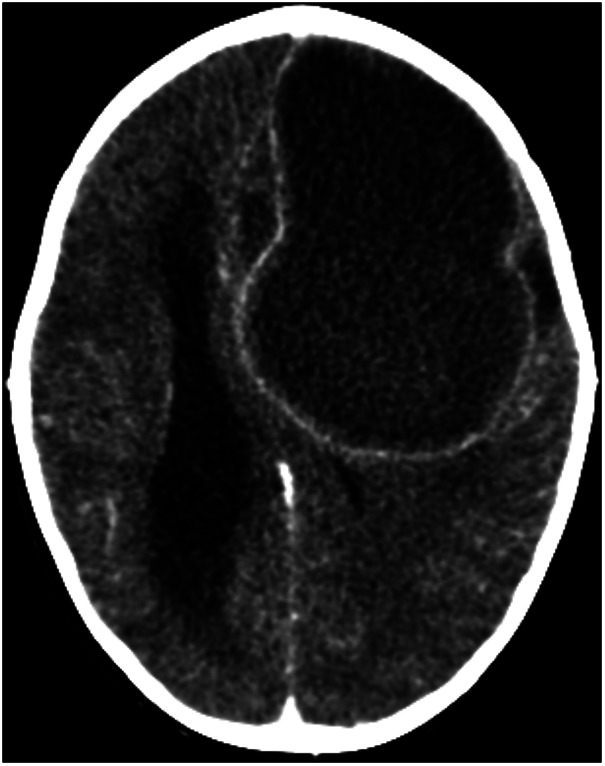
Initial contrast-enhanced cranial computed tomography showing a cerebral abscess. Axial contrast-enhanced cranial computed tomography demonstrates a large left frontotemporal hypodense lesion with peripheral ring enhancement, consistent with a cerebral abscess. Extensive surrounding vasogenic edema is observed, associated with subfalcine herniation and significant midline shift toward the right hemisphere. Compression of the ipsilateral lateral ventricle is also evident.
